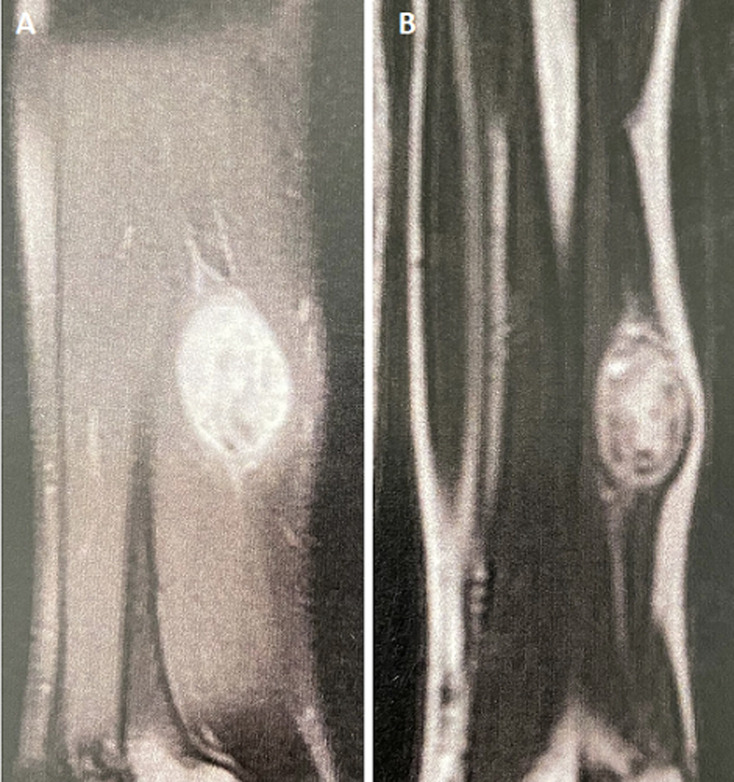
A,B) magnetic resonance imaging (MRI) aspect: soft tissue process well delimited and pseudo-encapsulated in relation to the course of the radial nerve compatible with a schwannoma
